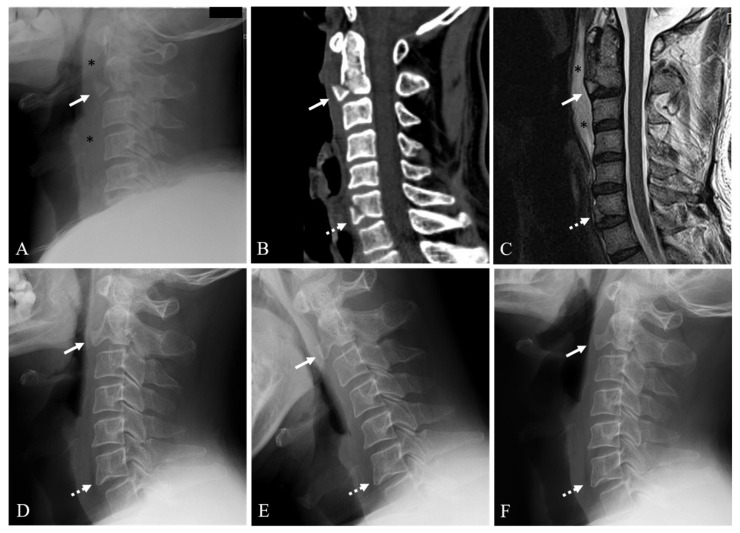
Lateral X-ray (A) and sagittal 2-dimensional (2-D) reconstructed computed tomography (CT) scan (B) showing anterior C2 tear drop (TD) fracture (white arrow), C6 fracture (dotted white arrow), and prevertebral soft tissue swelling (asterisks). Sagittal magnetic resonance imaging (C) showing anterior C2 TD fracture with 38% avulsion fracture ratio (white arrow), C6 fracture (dotted white arrow), and prevertebral hematoma (asterisks). At 12-month follow-up after Philadelphia brace, neutral (D), flexion (E), and extension (F) lateral X-rays showing solid fusion of anterior C2 TD fracture (white arrow) and C6 fracture (dotted white arrow).
